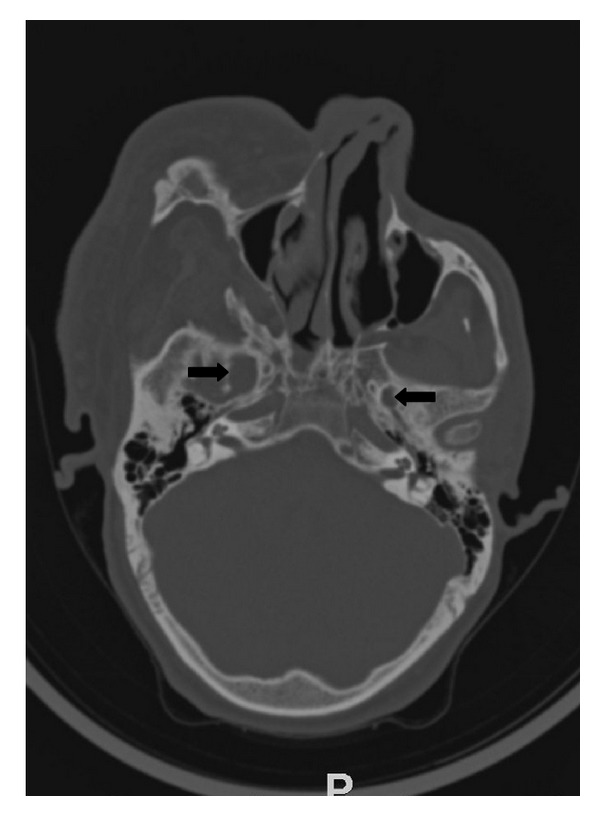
Axial CT scan with bone reconstructions showing widened foramen ovale (right side-arrow, left side-arrowhead).
